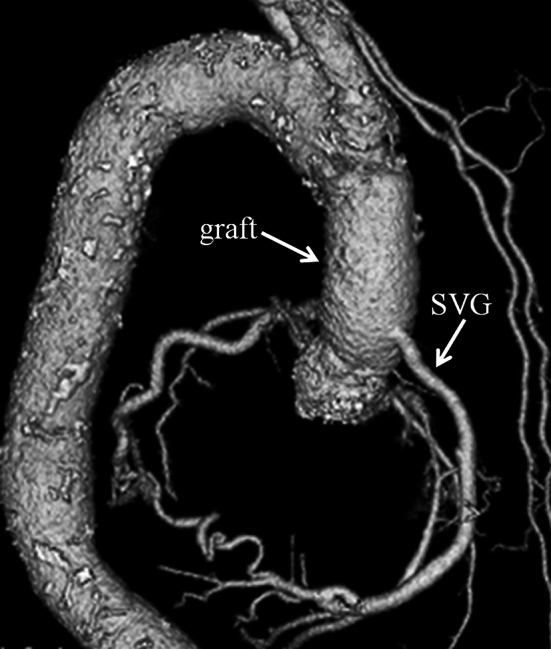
Post-operative 3D-CT image on the posterior view demonstrating well-enlarged aortic root, patent saphenous vein graft (SVG) and the ascending aorta replaced with a graft
